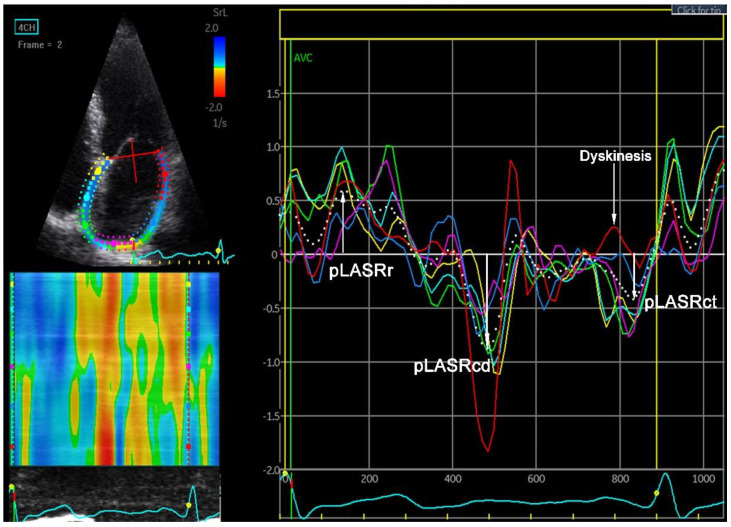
Left atrial wall dyskinesis. Stretching of one of the left atrial wall segments during the contractile phase. The phenomenon of left atrial wall dyskinesia is assessed during pLASRct; in the contractile phase, all segments of the left atrial wall should contract, while when dyskinesia occurs, one or several segments expand. The measurement was made in the apical four-chamber projection during sinus rhythm.
